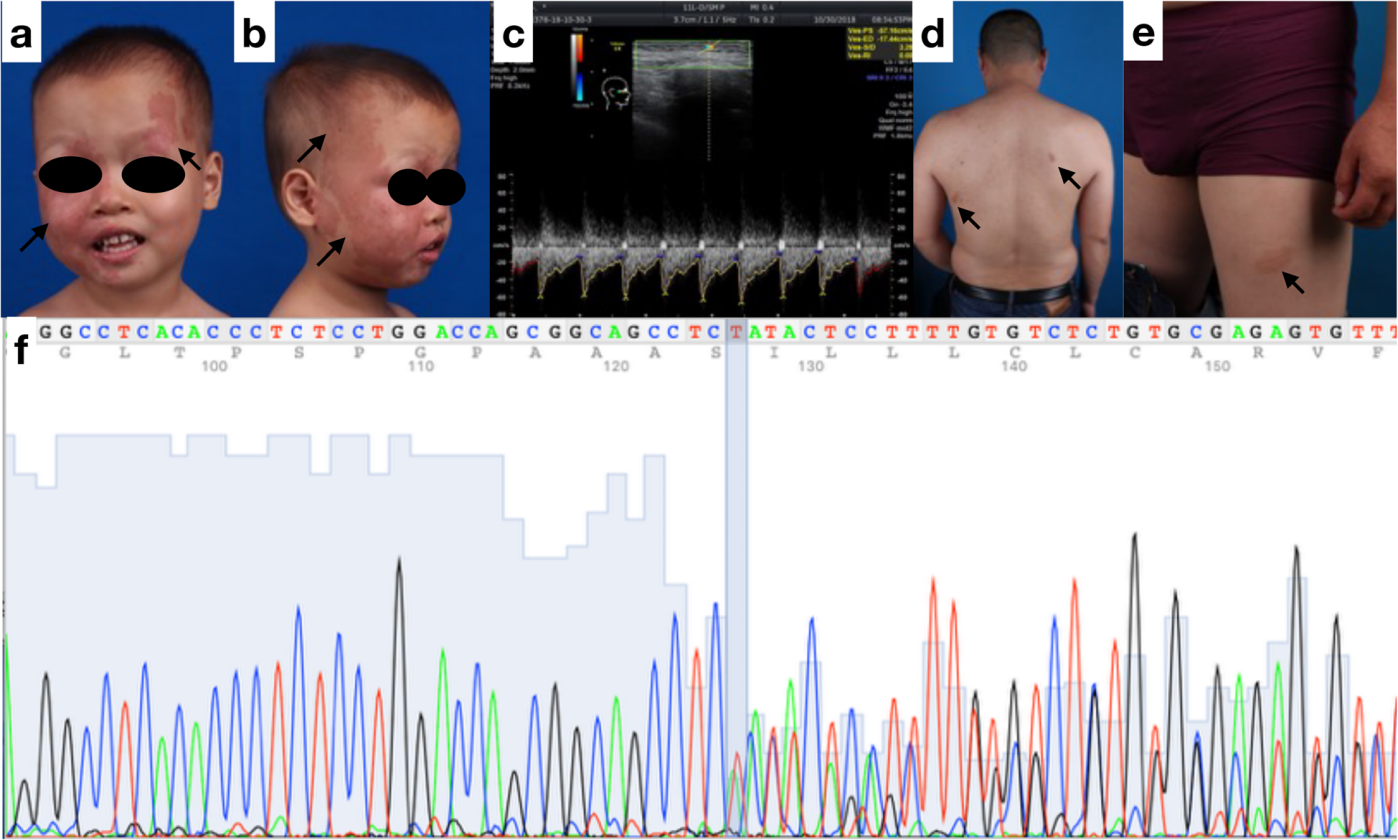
a-b Clinical manifestation of the patient. c Ultrasound scan of the patient in the facial lesions. d lesions in the back of proband’s father. e lesions in the leg of proband’s father. f Sanger sequence of germline mutation of EPHB4(7:100402906 AGT > A)
